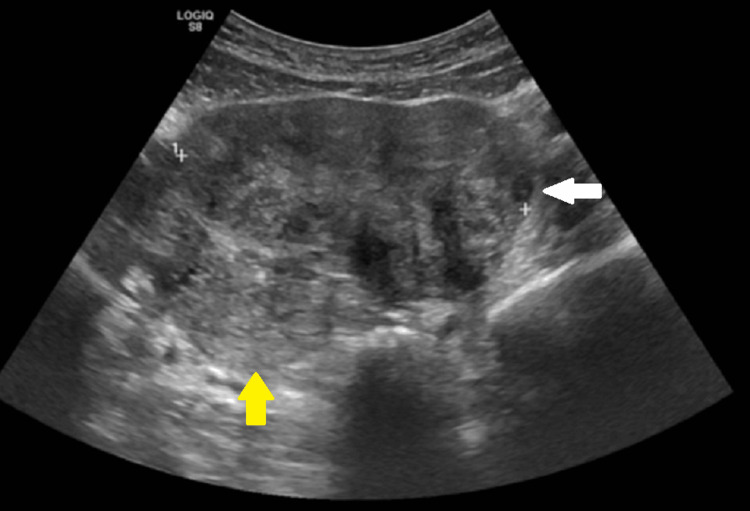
A large 11 x 10 cm mass (white arrowhead) with heterogeneous echotexture seen closely adjacent to the uterine fundus (yellow arrowheads) on ultrasonography
